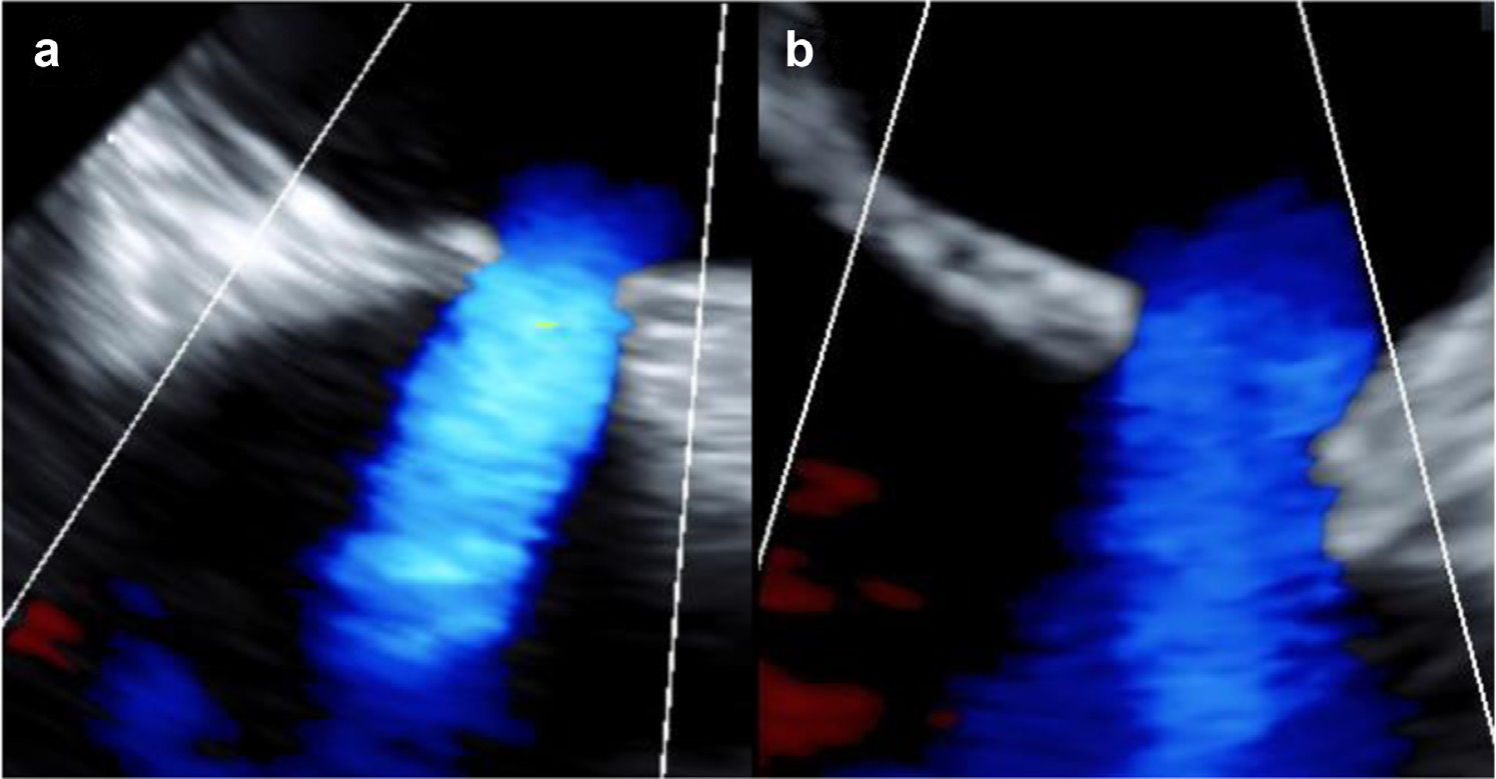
Representative images of transesophageal echocardiographic assessment of a human subject showing shunt patency sustained through 6-month follow-up. (a) Baseline, immediately post-shunt creation. (b) Six-month follow-up.
